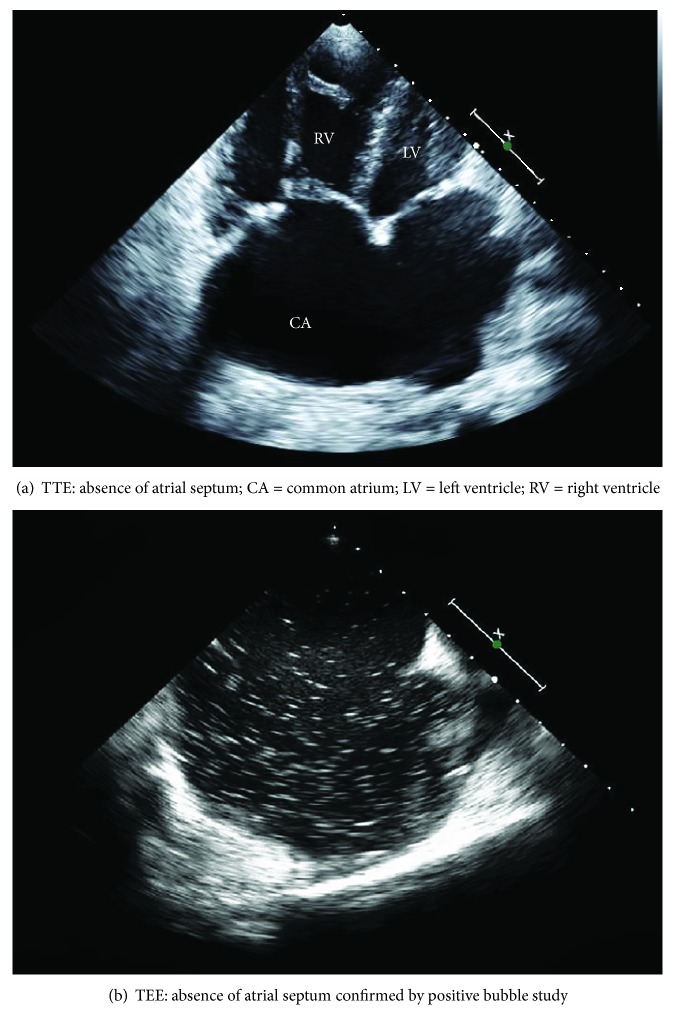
Echocardiography images revealing common atrium. (a) TTE: absence of interatrial septum. (b) TEE: positive bubble study.
